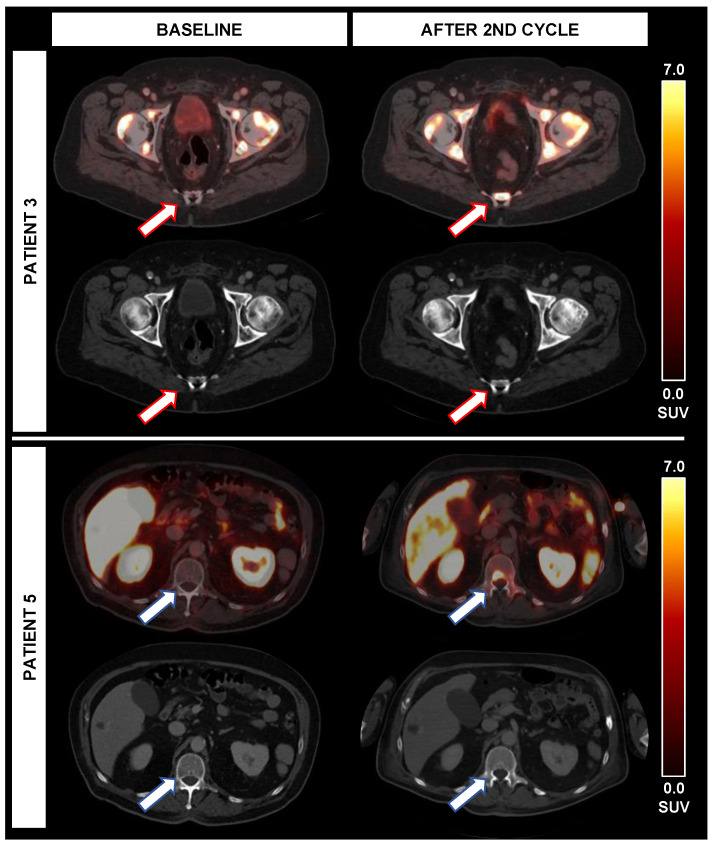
Occurrence of new PSMA-avid lesions during alpha therapy. While patient 3 experienced new PSMA-avid lesions (red arrows) without correlate on CT, the TTV (+3%) was stable and PSA (−33%) was decreasing after 2 cycles. In patient 5, new bone lesions (blue arrow) and lymph nodes with PSMA-expression were noted after the second cycle; however, both TTV (−65%) and PSA (−25%) were decreasing despite new lesions.
